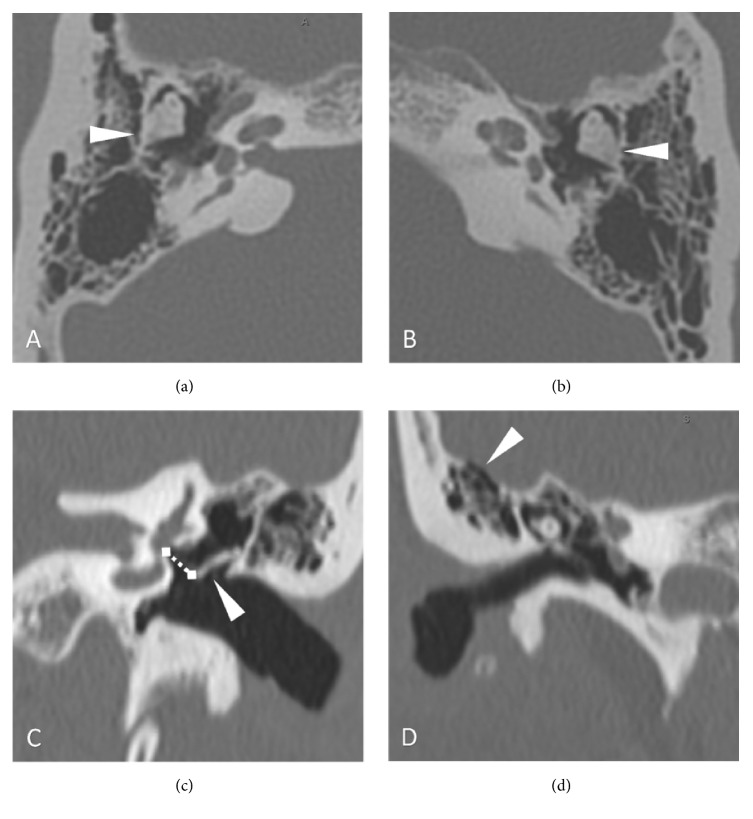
Axial CT bone windows of the temporal bones revealed a markedly enlarged incus with possible fixation in both the right (a) and the left ears (a). Coronal reformats on the left (c) demonstrated an elongated stapes superstructure of approximately 4.8 mm (dashed line) with thickening of the incus long process (arrowhead). On the right (d), this measurement was 4.5 mm. Coronal views of the right ear demonstrated bony dehiscence or thinning in the tegmen mastoideum without meningoencephalic herniation. Similar findings were noted on the left.
